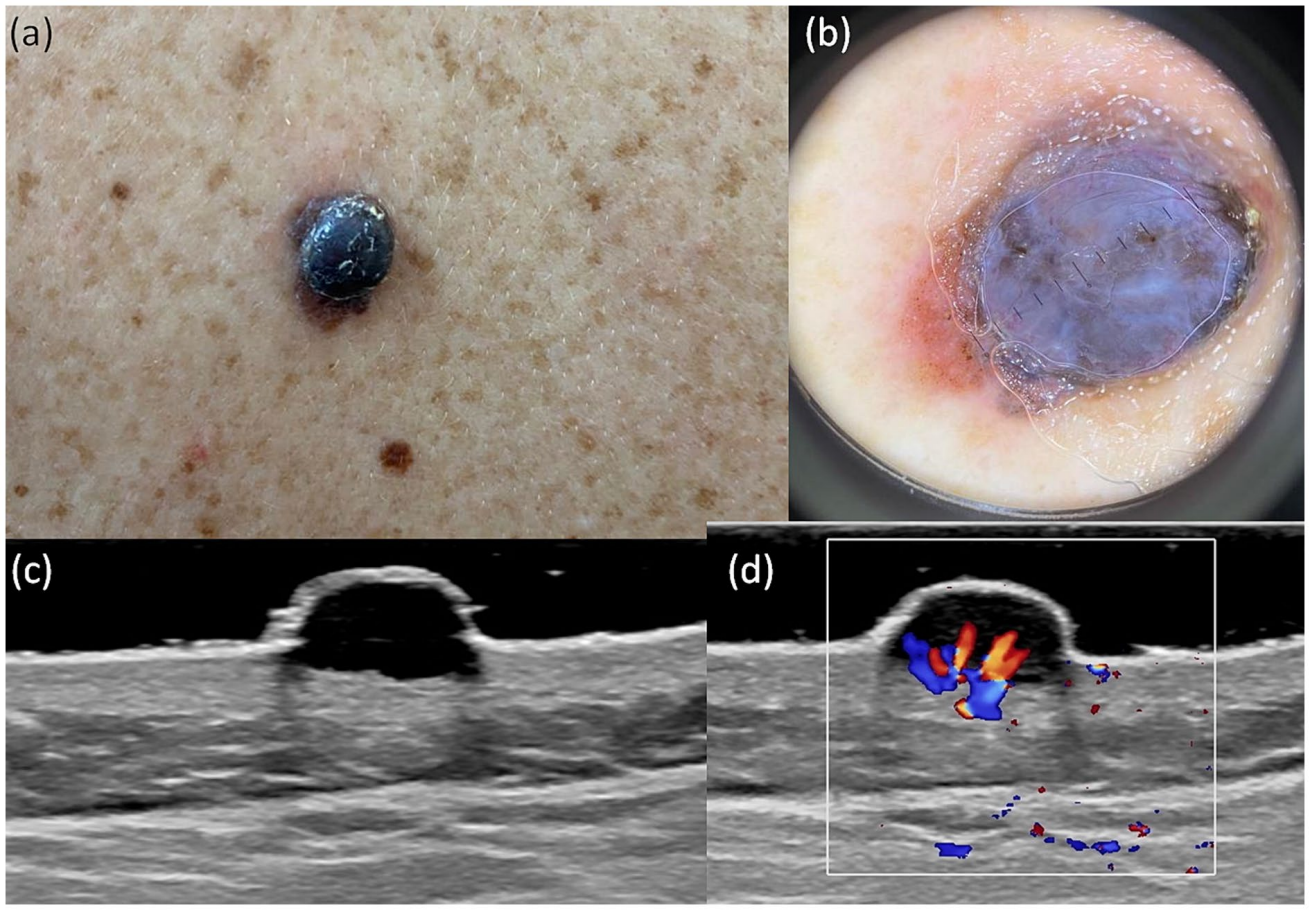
Clinical picture of MN (A). Dermatoscopy shows the presence of a nodular tumor with blue–white veil, with atypical vessels on surface, and blue–black color (B). US shows a hypoechoic, oval, well-defined lesion that reaches the level of the reticular dermis (C). Doppler mode shows an increase in tumor vascularity (D).
